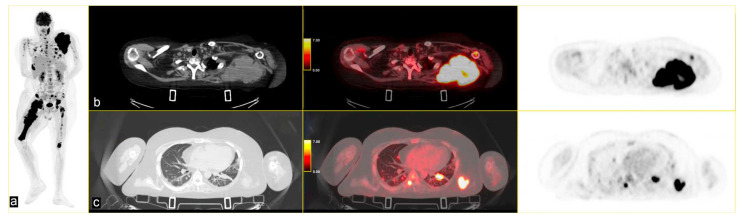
(a) MIP PET/CT showing significant disease progression; (b) volumetric and metabolic increase of the lesion in the left scapula (SUV_max_ 35); and (c) multiple lesions in the upper lobes of both lungs (SUVmax 25.3) on (b,c) strips CT on the left, PET/CT fused images the middle and PET images on the right.
